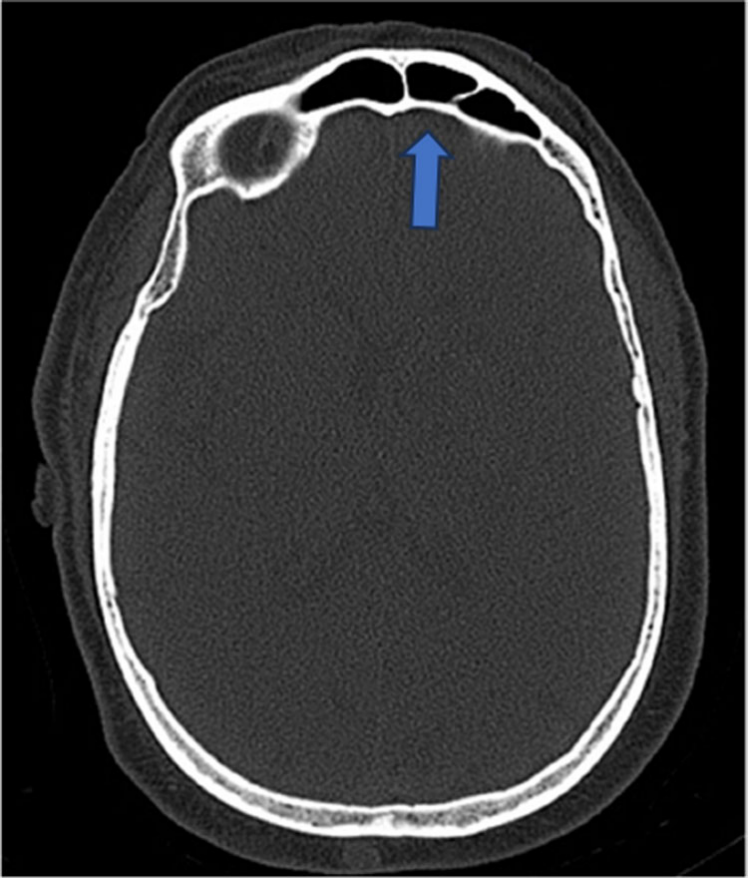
CT axial image shows more than two chambers of frontal sinus.
